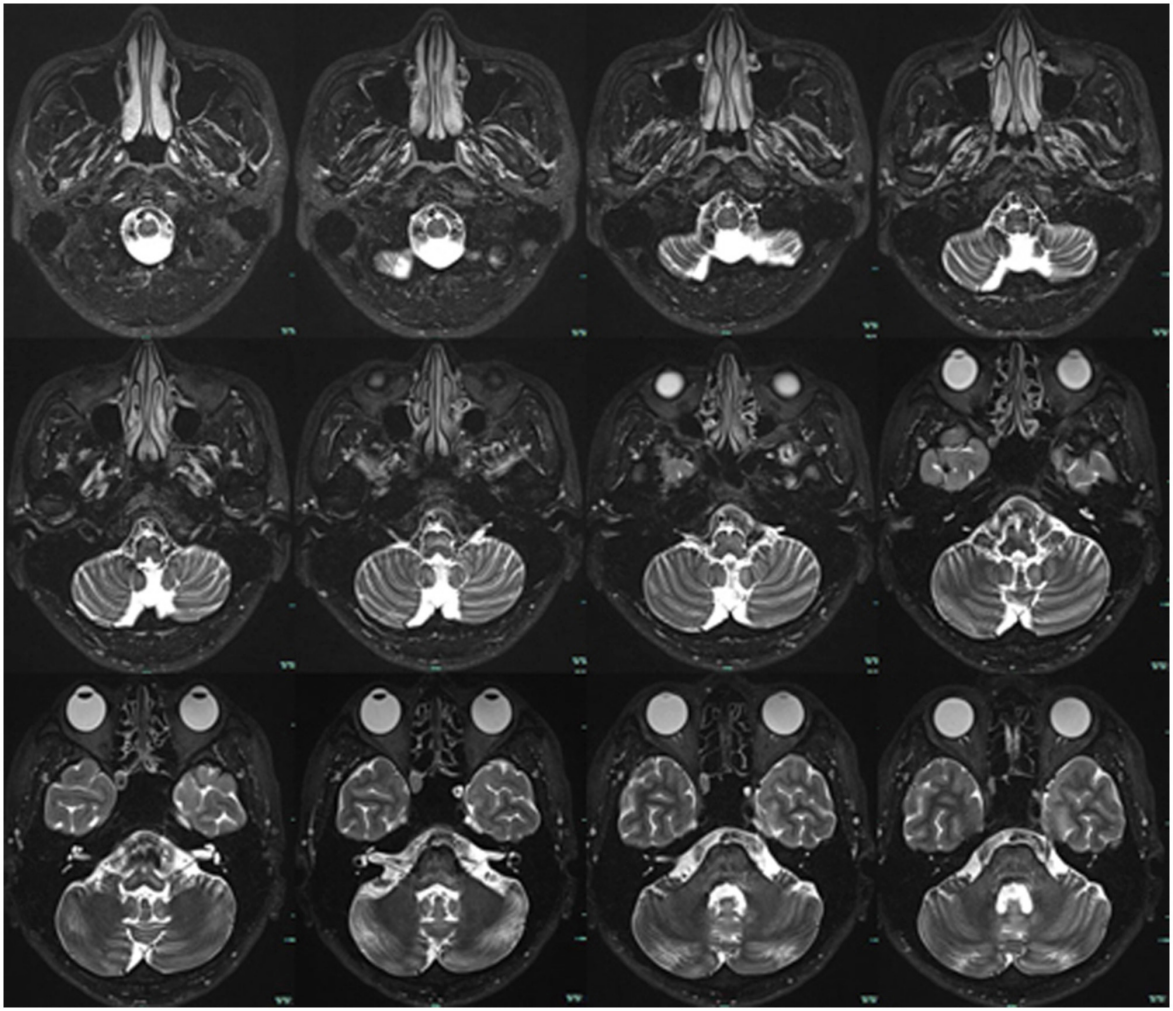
Brain MRI. T2-weighted sequences showed no tumor or other lesions in the cerebello-pontine angle and brainstem.
